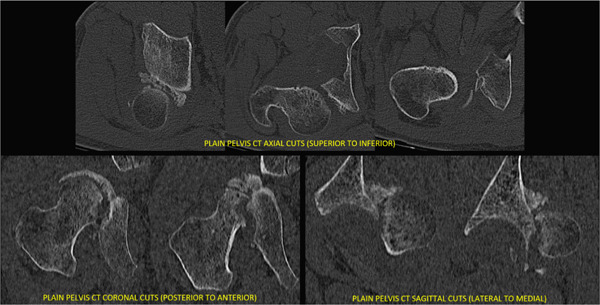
Case 5 preoperative computed tomography.
